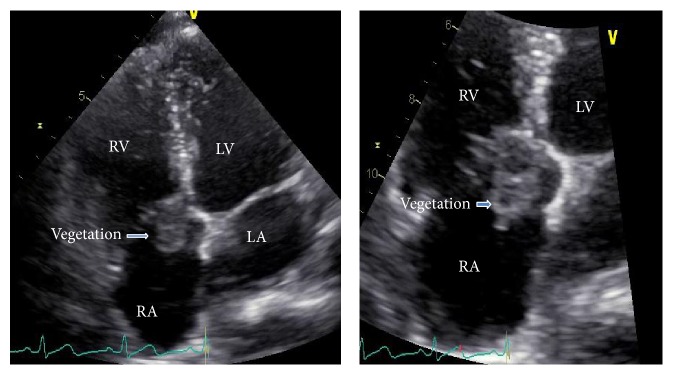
Transthoracic echocardiogram: four chambers' view of the heart showing large vegetation attached at tricuspid valve along right atrial surface. RA: right atrium; LA: left atrium; RV: right ventricle; LV: left ventricle.
